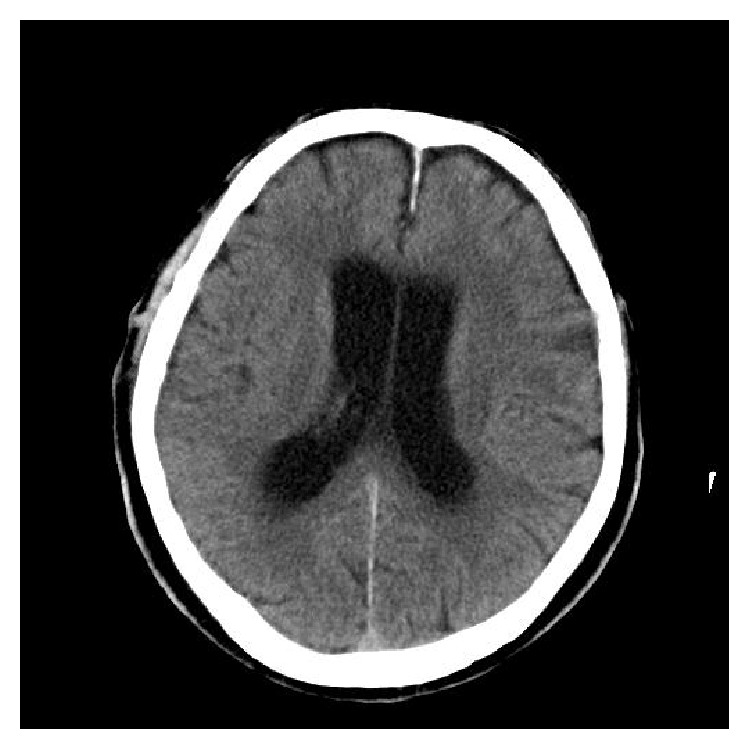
CT of the brain without intravenous contrast on the fifth day of admission showing development of a communicating hydrocephalus.
